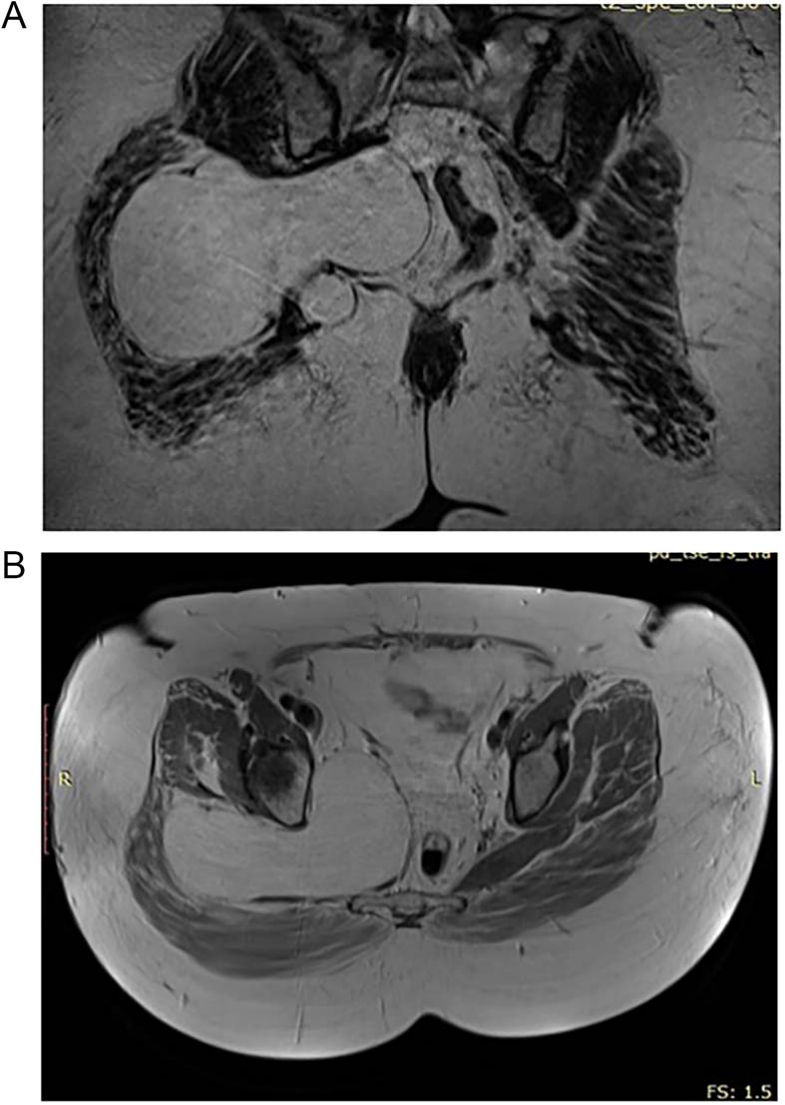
(A) Preoperative CT of the 55 year old patient with lipoma in the pelvic region. (B) Preoperative CT of the 55 year old patient with lipoma in the pelvic region.
